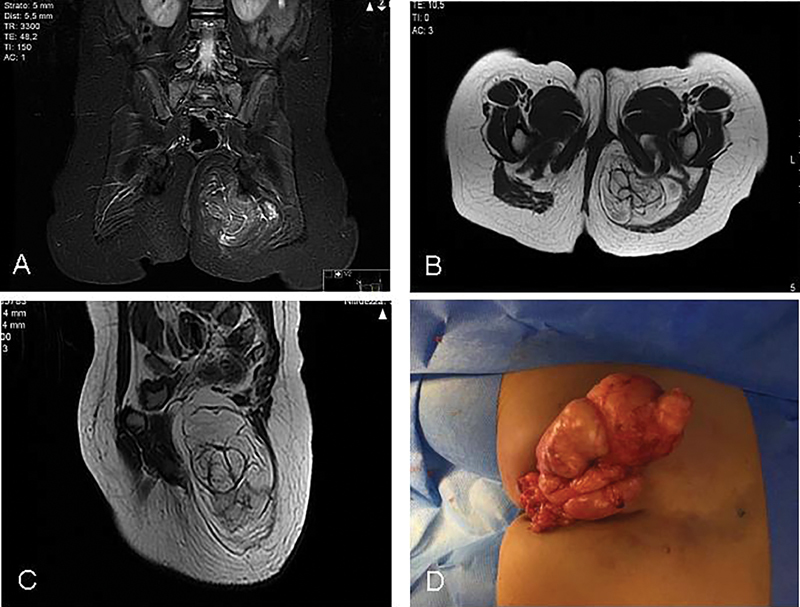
Magnetic resonance imaging showed a multilobulated epifascial lesion measuring 8 × 6 cm in the context of the gluteal muscle that deepened in the pelvis (
